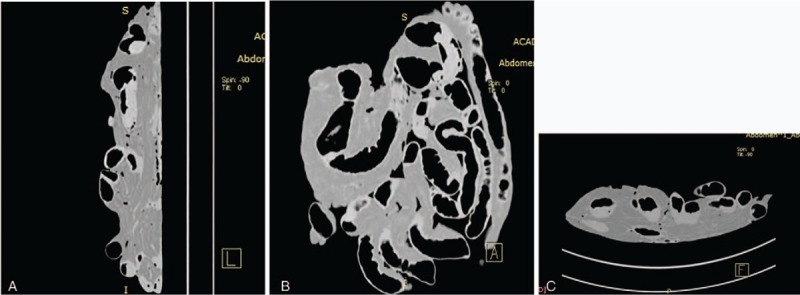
Coronal, sagittal, and cross-sectional screenshots of air-enhanced computed tomography images.
